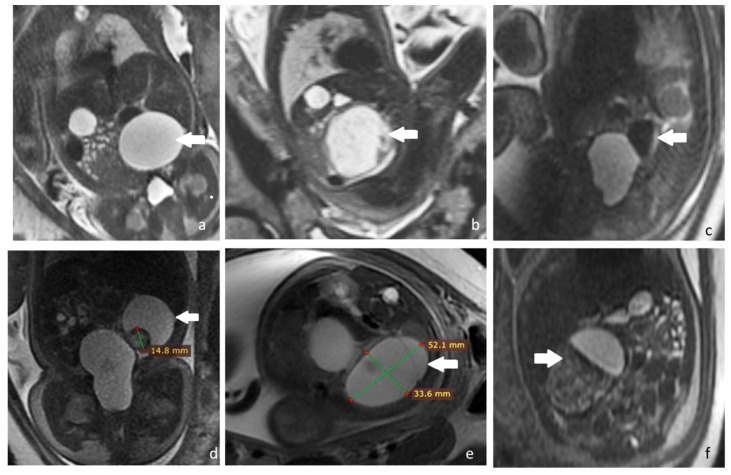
Fetal ovarian cyst-MRI aspects. All images are T2 SSFSE (single shot fast spin echo): (a) Simple ovarian cyst. Coronal view with a fluid filled cyst (arrow); (b) hemorrhagic cyst. Sagittal view with an inhomogeneous cyst, with hypointense fluid-fluid level (arrow); (c) hemorrhagic cyst. Sagittal view showing a cyst with hemorrhagic hypointense content and fluid-fluid level (arow); (d) daughter cyst. Coronal view showing a hemorrhagic daughter cyst in a larger fluid filled cyst (arrow); (e) septated ovarian cyst. Axial view showing a cyst with fine internal septations and uncomplicated fluid hyperintensity (arrow); (f) ovarian torsion. Enlarged ovary with inhomogeneous structure and a fluid-filled cyst, mimicking a fluid-fluid level (arrow).
